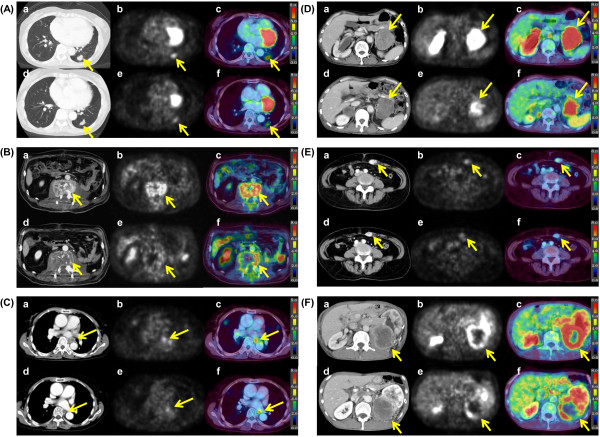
FDG PET/CT features in the pretreatment state and post-treatment state. CT imaging (a), PET imaging (b) and fused imaging (c) before treatment and CT imaging (d), PET imaging (e) and fused imaging (f) at1 month after TKI treatment initiation. A: A 55-year-old female with lung metastasis. She was treated with sorafenib. The SUVmax showed a 25.6% decrease (from 4.3 to 3.2) and the tumor dia. showed a 13.6% decrease. B: A 66-year-old male with lumbar vertebrae metastasis. He was treated with sunitinib. The SUVmax showed a 27.5% decrease (from 8.0 to 5.8) and the tumor dia. showed a 3.9% decrease. C: A 76-year-old male with mediastinal lymph node metastasis. He was treated with sorafenib. The SUVmax showed a 13.2% decrease (5.3 to 4.6) and the dia. showed a 10.0% decrease. D: A 64-year-old male with adrenal gland metastasis. He was treated with sunitinib. The SUVmax showed a 25.0% decrease (8.8 to 6.6) and the dia. a 8.5% decrease. E: A 67-year-old female with rectus abdominis muscle metastasis. She was treated with sorafenib. The SUVmax showed a 26.5% decrease (3.4 to 2.5) and the dia. a 16.0% decrease. F: A 71-year-old male with primary tumor in the left kidney. He was treated with sunitinib. The SUVmax showed a 21.4% decrease (8.4 to 6.6) and the dia. did not change.
